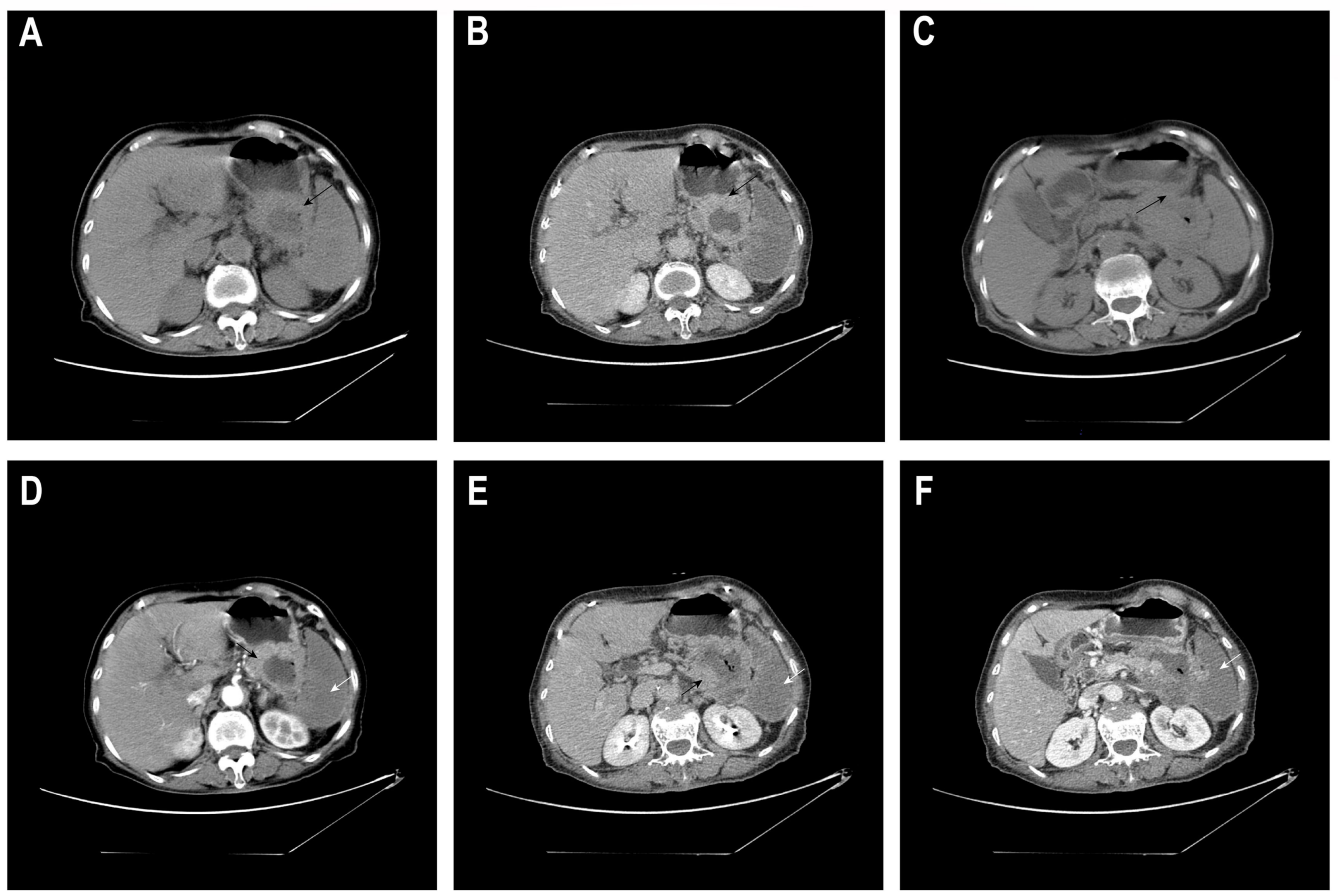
Abdominal CT plain scan plus enhancement. The same axial slice 1 (A, D, B, E): (A, plain scan) A space-occupying lesion in the space between the spleen and stomach (black arrow ←); (D, arterial phase) The lesion shows significant enhancement at the margin, accompanied by patchy non-enhanced areas in the spleen (white arrow ←); (B, venous phase) The lesion shows moderate to high enhancement at the margin (black arrow ←), while the low-density area inside remains non-enhanced; (E, equilibrium phase) The spleen is full, with large areas of low/no enhancement (white arrow ←). The same axial slice 2 (C, F): (C, plain scan) A low-density space-occupying lesion in the space between the spleen and stomach, with unclear boundaries from the adjacent posterior wall of the stomach, spleen, pancreas and part of the intestinal tract, seemingly communicating with the gastric cavity (black arrow ←); (F, venous phase) Large areas of low/no enhancement in the spleen (white arrow ←).
